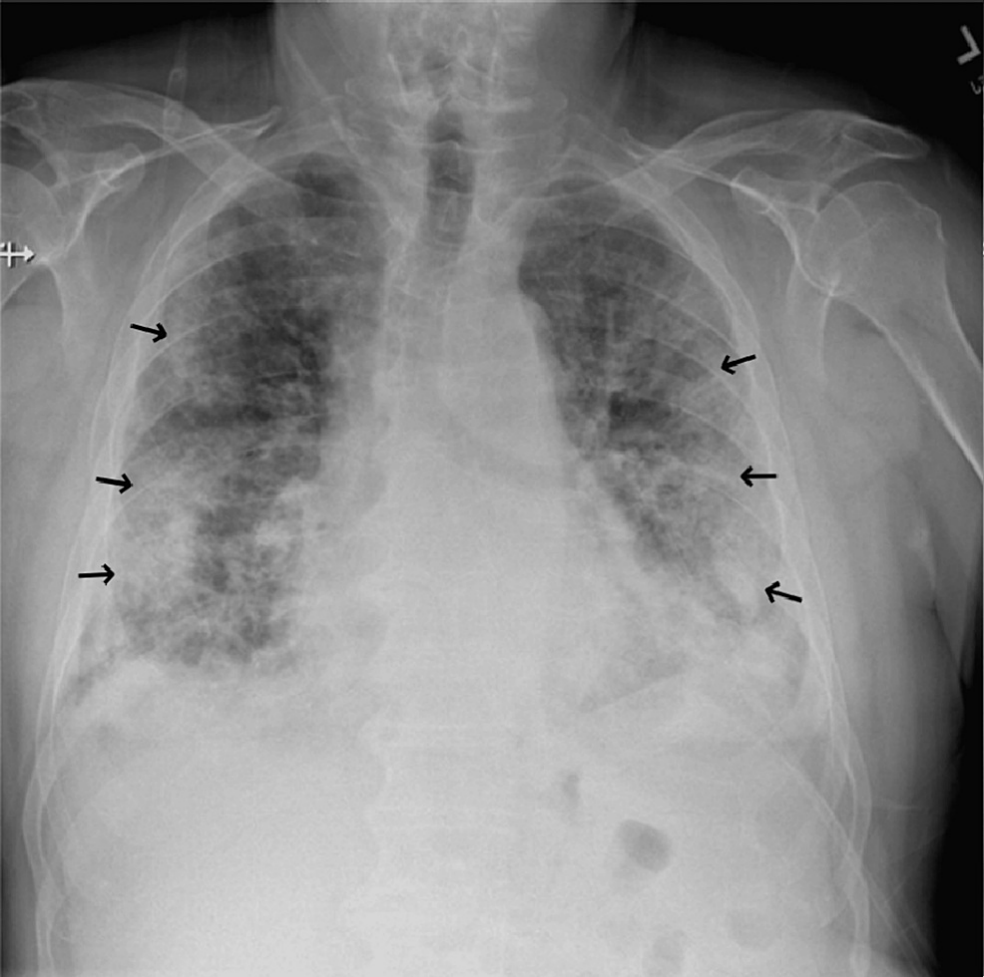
Posterior-anterior chest X-ray on arrival showing bilateral parenchymal infiltrates
